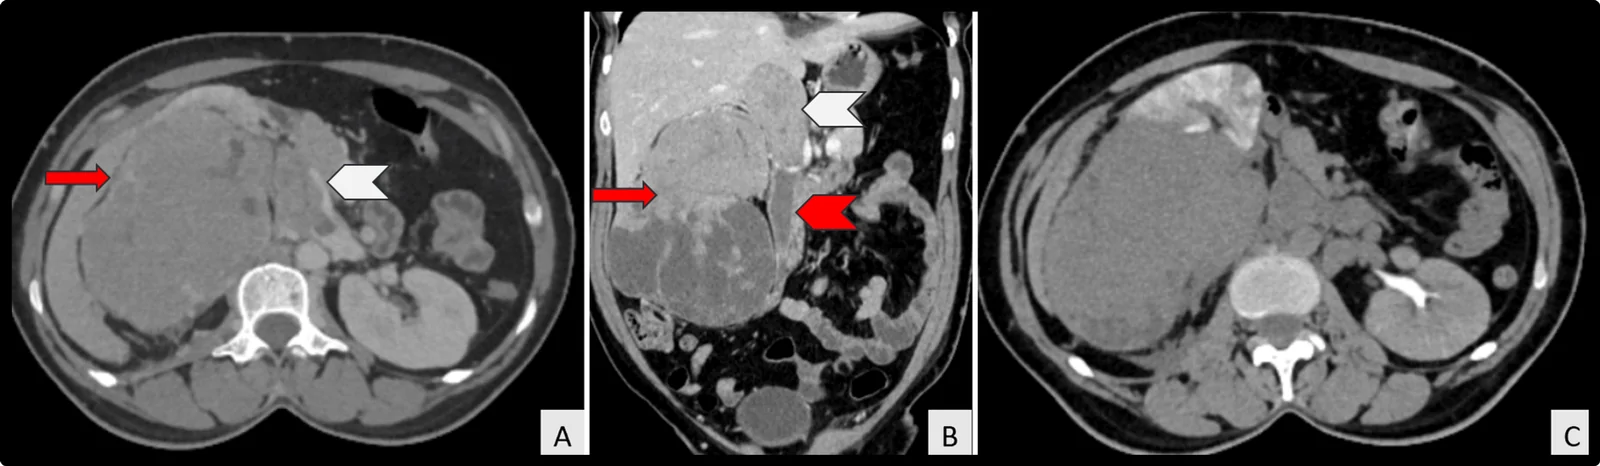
CECT urography demonstrating the primary renal mass and venous involvement A: Axial nephrographic phase of CT urography image demonstrates a large heterogeneously enhancing right renal mass (red arrow) with contiguous tumor thrombus extending into the right renal vein and inferior vena cava (IVC) (white arrowhead). B: Coronal reconstructed CT urography image showing mass lesion (red arrow) and extension of the enhancing thrombus from the right renal vein into the IVC (white arrowhead), as well as non-enhancing bland thrombus in the rest of the IVC (red arrowhead). C: Delayed excretory-phase CT urography image demonstrates absence of contrast excretion from the right kidney, consistent with a non-functioning obstructed kidney secondary to extensive tumor involvement. CECT: Contrast-enhanced computed tomography
